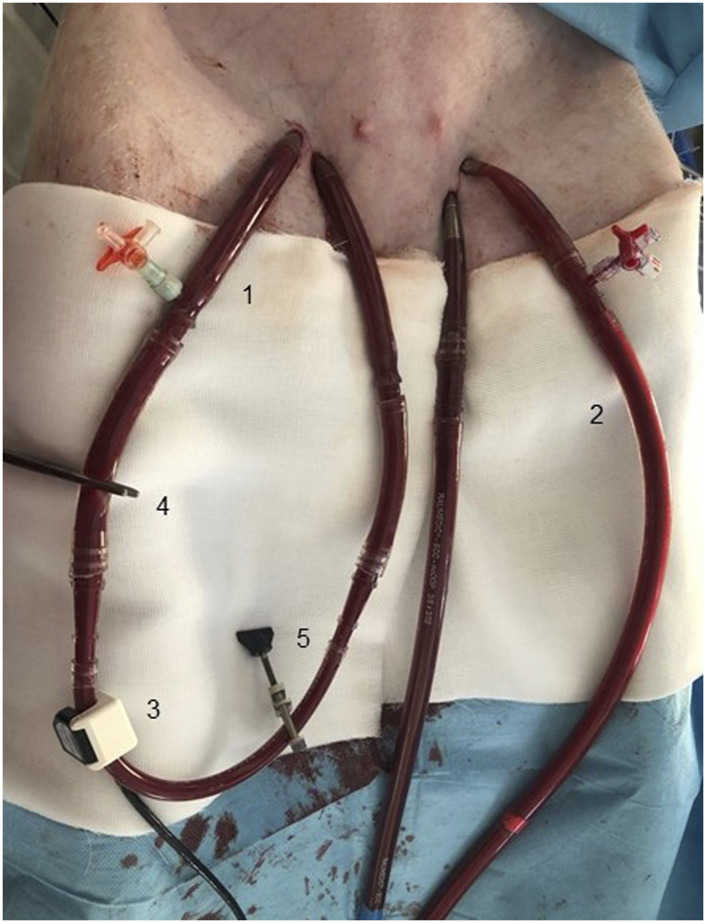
Insertion of ECMO cannulas for V-A ECMO support and to create an AVF. Arteriovenous fistula was created using two ECMO cannulas connected by a plastic tube 1). Two cannulas inserted in contralateral femoral vessels were used for connection to the ECMO circuit 2). AVF and ECMO flow were measured using an ultrasound probe 3). Hemostatic forceps was used to close/open the arteriovenous fistula 4). AVF blood flow was regulated using a special clamp 5).
